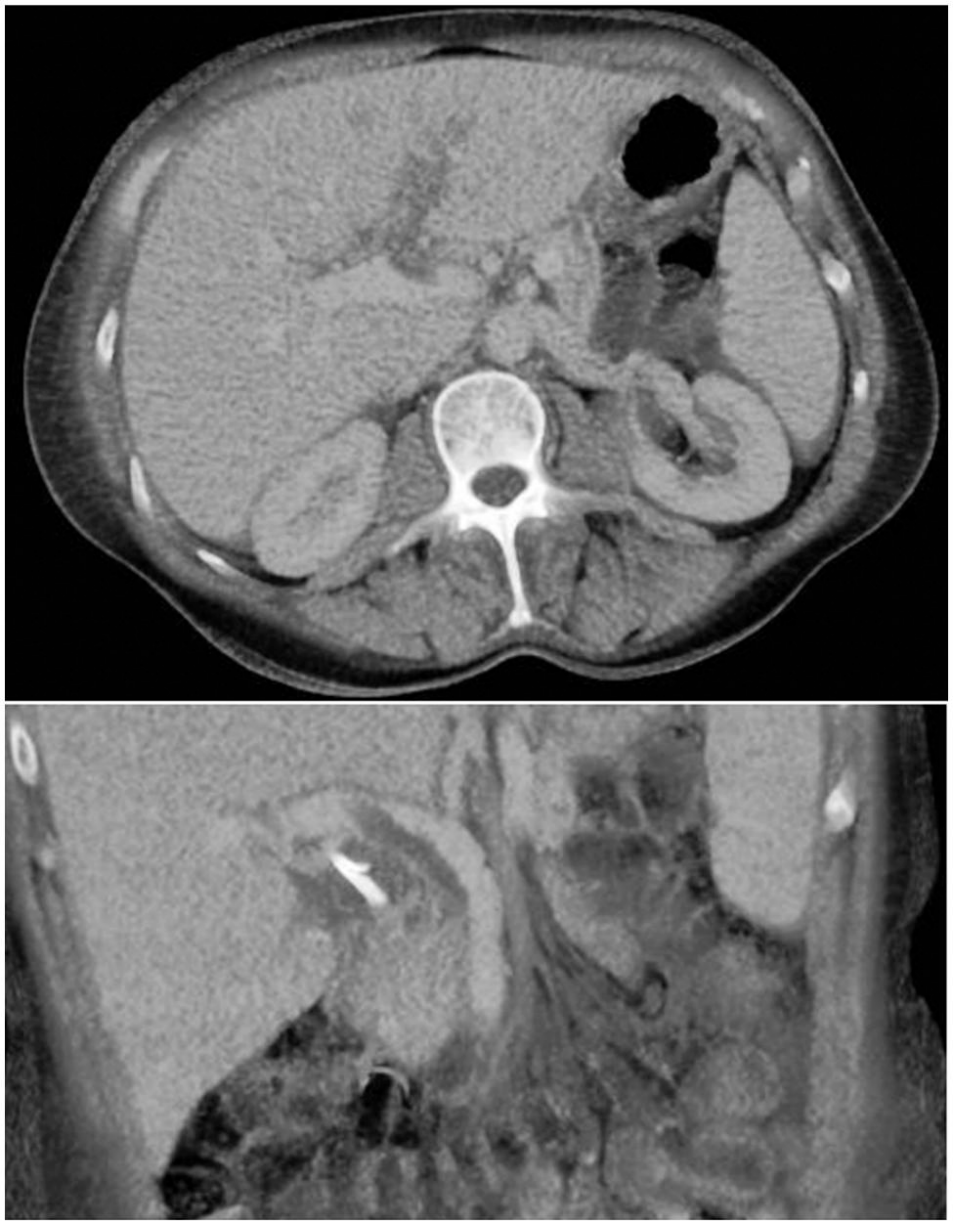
Portal vein thrombus extending into the left portal vein. Portal venous contrast-enhanced CT with maximal intensity projection (MIP) images demonstrates partially occlusive portal vein thrombus extending into left portal vein. Common bile duct and pancreatic stents are *in situ*.
